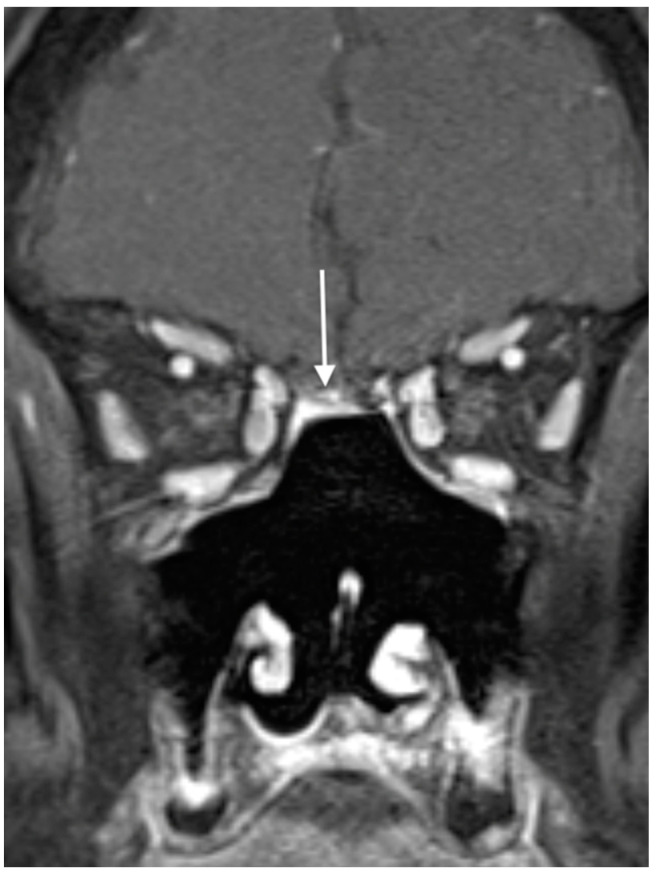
Magnetic resonance imaging for the surveillance of olfactory neuroblastoma. A postresection cavity is seen here without evidence of recurrent disease. The white arrow indicates persistent enhancement of a pedicled nasoseptal flap used in reconstruction.
